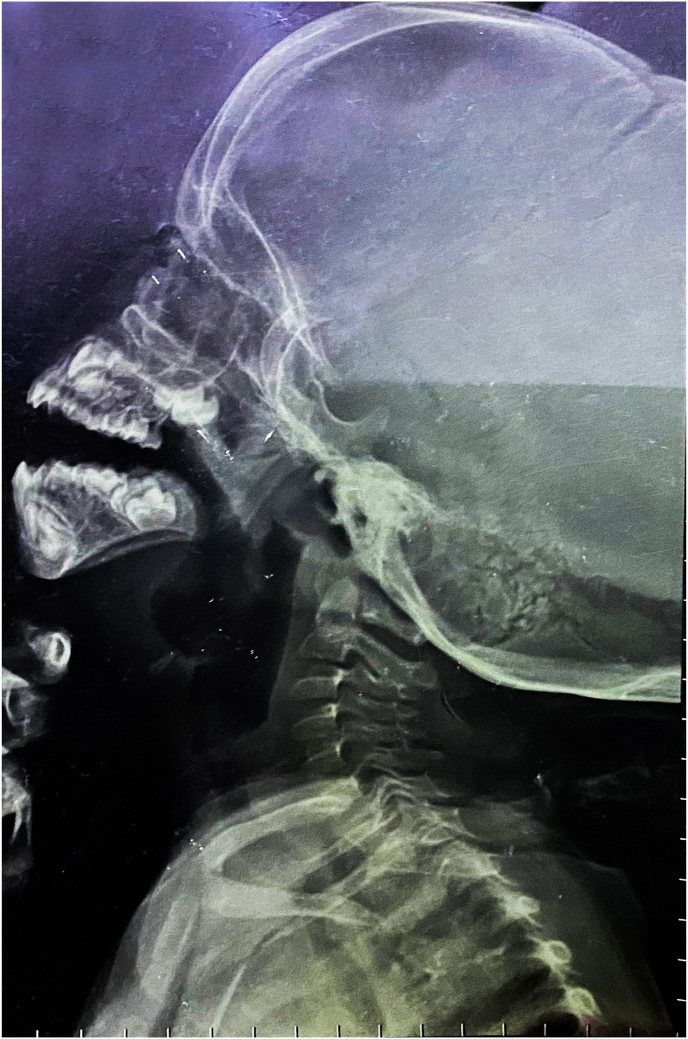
Head and neck x-ray showing elongated sella turcica and an elevated palate.
